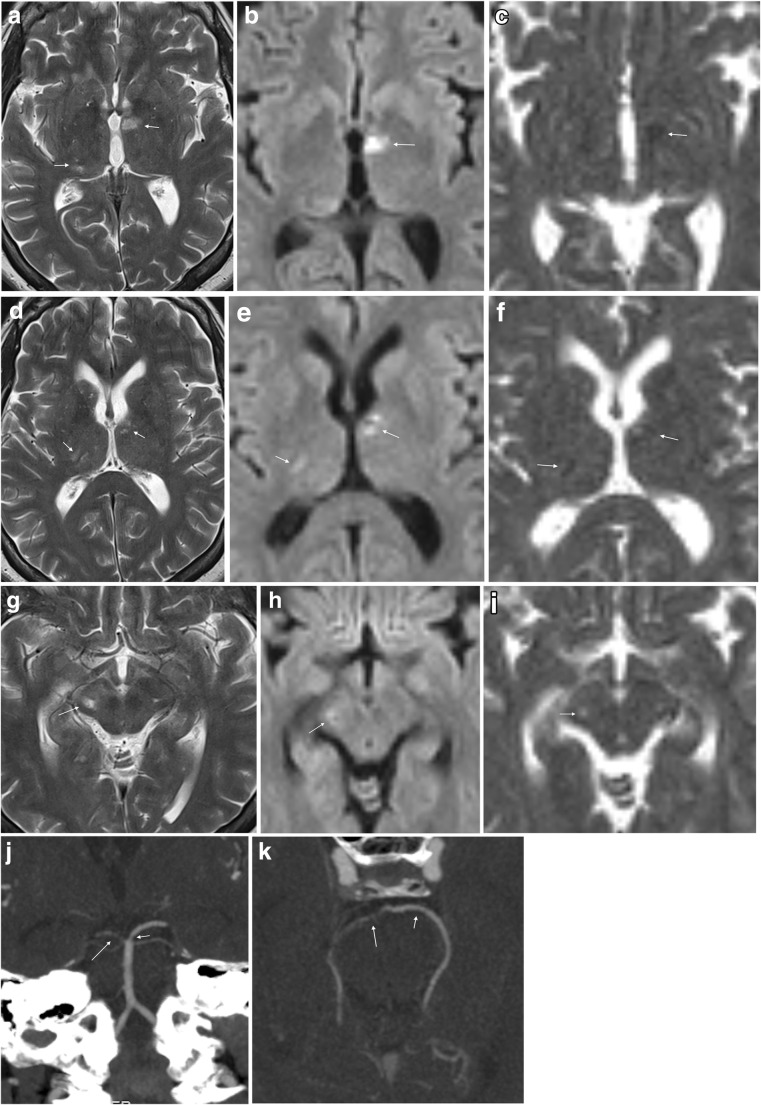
LNB vasculitis in a 55-year-old woman with strange behaviour for 3 days preceding hospital admission. She had incoherent speech and was disorientated. CSF cell count was 213 cells/mm^3^ and *Bb* IgG AI was positive. MRI revealed ischemic lesions of different ages in thalamus bilaterally (a–f), right cerebral peduncle (g–i) and right occipital cortex (not shown). Axial T2-weighted images (a, d, g), diffusion weighted B1000 images (b, e, h) and ADC maps (c, f, i) demonstrated the lesions. In contrast to the hypointense lesions on ADC maps in the thalami (arrows in c and f) consistent with cytotoxic edema in recent infarcts, the lesion in the right cerebral peduncle was hyperintense on both B1000 image (arrow in h) and ADC map (arrow in i), consistent with vasogenic edema and a more advanced stage of ischemic lesion. 5 mm MIP coronal (j) and axial (k) reformats of CT angiography examination demonstrated several short stenotic segments of both posterior cereberal arteries (arrows), most pronounced on the right side
